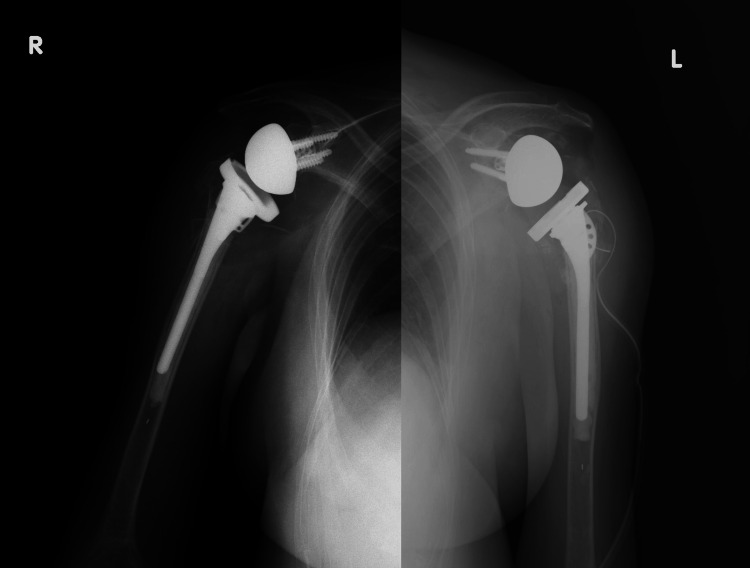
Postoperative radiographs following bilateral reverse shoulder arthroplasty Postoperative anteroposterior X-ray images of both shoulders after reverse shoulder arthroplasty.
